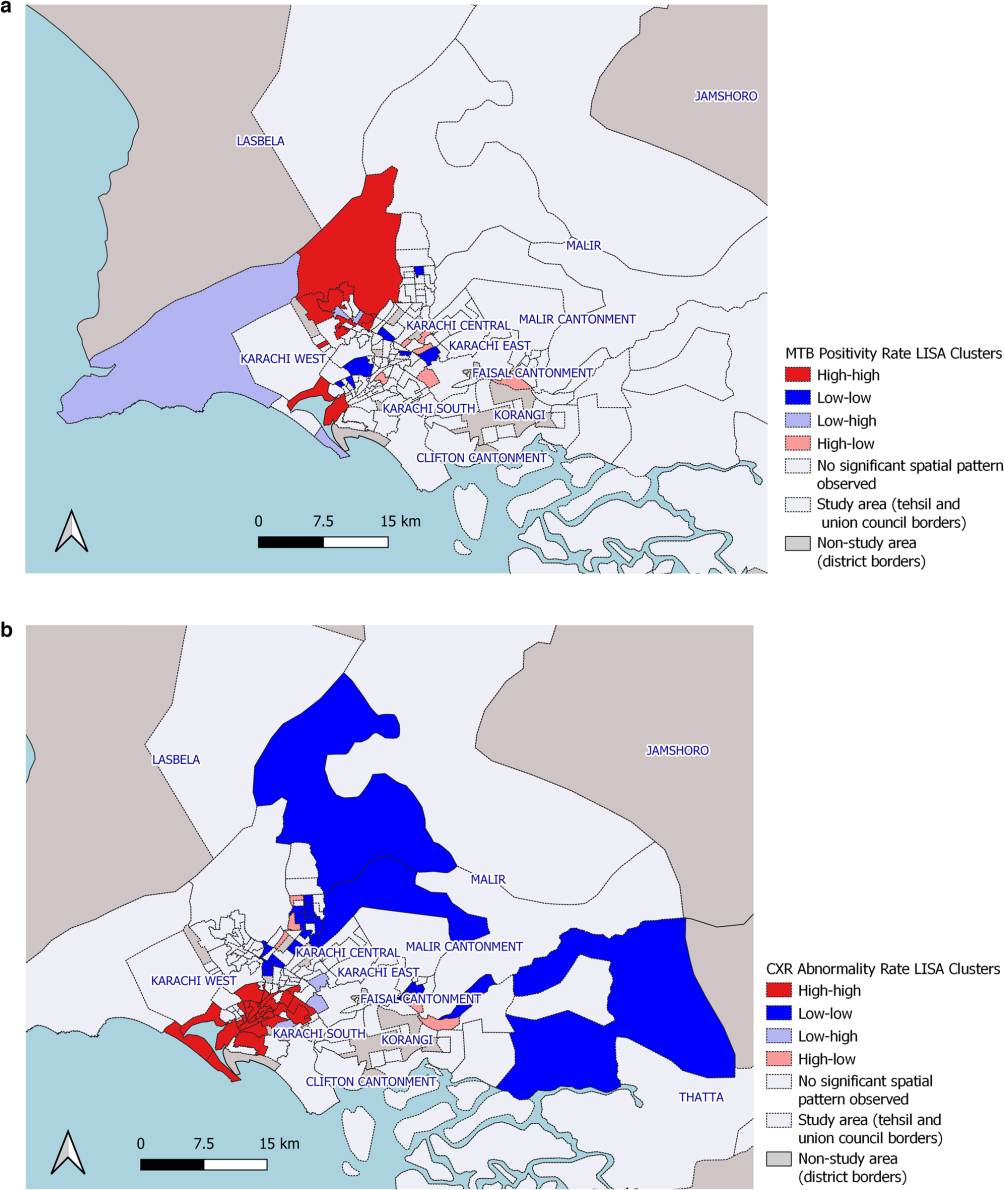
(a) Local Indicators of Spatial Association (LISA) analysis for MTB positivity rates in Union Councils (UCs) from mobile chest X-ray supported active case-finding camps in Karachi, Pakistan (July 2018–March 2020). A High-high result indicates potential clustering of UCs with high MTB positivity. A High-low result indicates a UC with a potentially high MTB positivity surrounded by UCs with low positivity. A low–low result indicates clustering of UCs with potentially low MTB positivity. A low–high result indicates a UC with potentially low MTB positivity surrounded by UCs with high positivity. QGIS Geographic Information System v3.26.3. QGIS.org, 2022. QGIS Association. http://www.qgis.org. (b) Local Indicators of Spatial Association (LISA) analysis for abnormal X-ray rates in Union Councils (UCs) from mobile chest X-ray supported active case-finding camps in Karachi, Pakistan (July 2018–March 2020). A High-high result indicates clustering of potential UCs with high abnormal X-ray rates. A High-low result indicates a UC with potentially high abnormal X-ray ratio surrounded by UCs with low rates. A low–low result indicates clustering of UCs with low abnormal X-ray rates. A low–high result indicates a UC with potentially low MTB positivity surrounded by UCs with high positivity. QGIS Geographic Information System v3.26.3. QGIS.org, 2022. QGIS Association. http://www.qgis.org.
